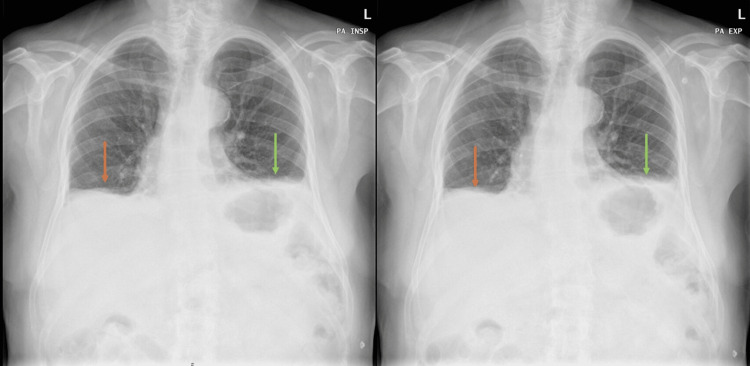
Chest radiographs during inspiration and expiration Absence of positional variation of the diaphragm on chest radiographs during inspiration (left) and expiration (right). The colored arrows demonstrate the absence of positional variation of the diaphragm.
